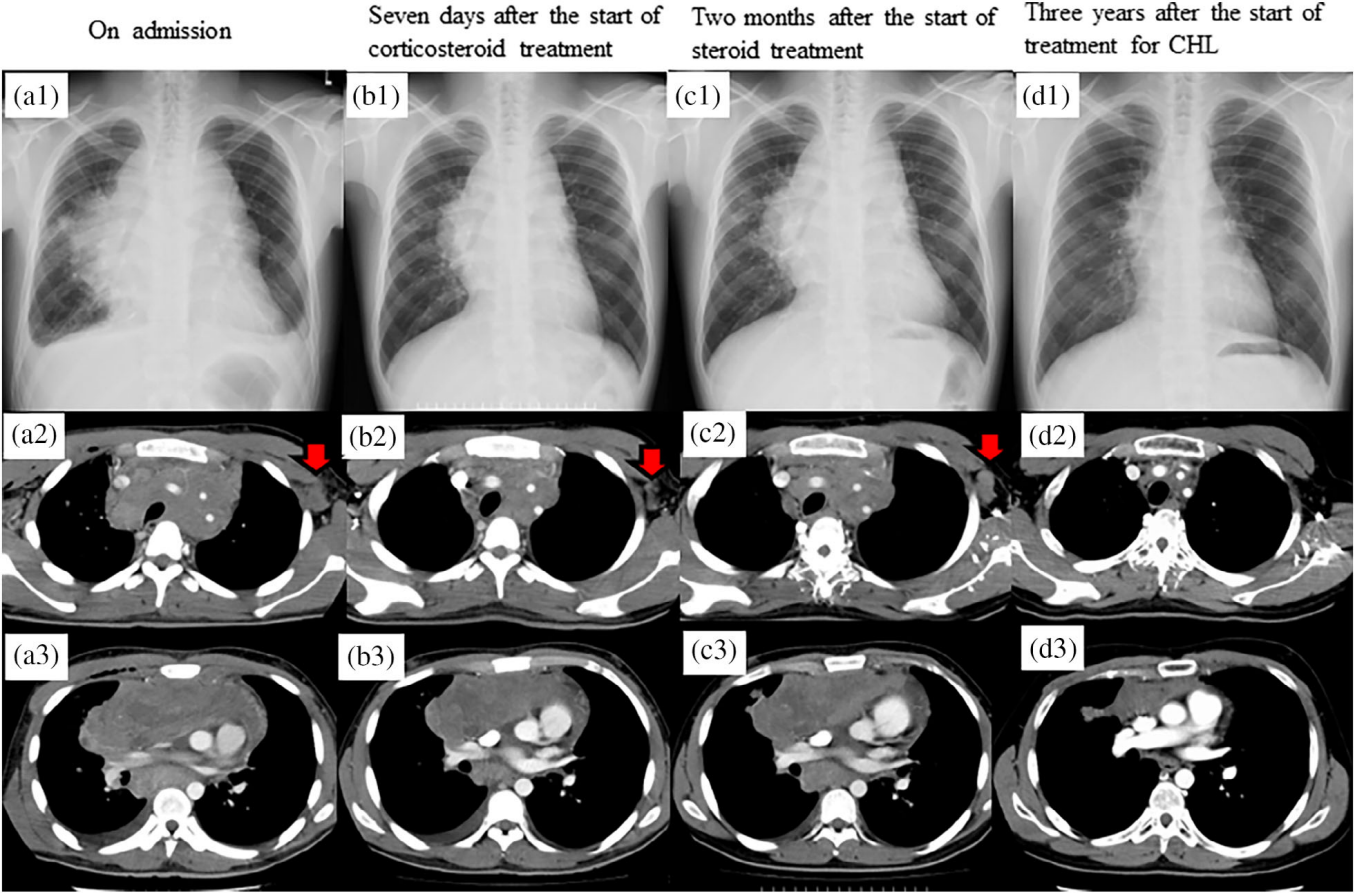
(a) Chest x‐ray film (a1) and computed tomography (CT) images (a2 and a3) on admission, showing a large mediastinal mass with pericardial and bilateral pleural effusions and left axillary lymphadenopathy (red arrow). (b) Chest x‐ray (b1) and CT images (b2 and b3) seven days after the start of corticosteroid treatment, showing shrinkage of the mediastinal tumor and lymph nodes (red arrow). (c) Chest x‐ray film (c1) and CT images (c2 and c3) two months after the start of corticosteroid treatment, showing regrowth of the mediastinal tumor and lymph nodes (red arrow). (d) Chest x‐ray film (d1) and CT images (d2 and d3) three years after the start of treatment for CHL, showing mediastinal tumor shrinkage and lymph node enlargement disappearance. CHL, classical Hodgkin lymphoma.
